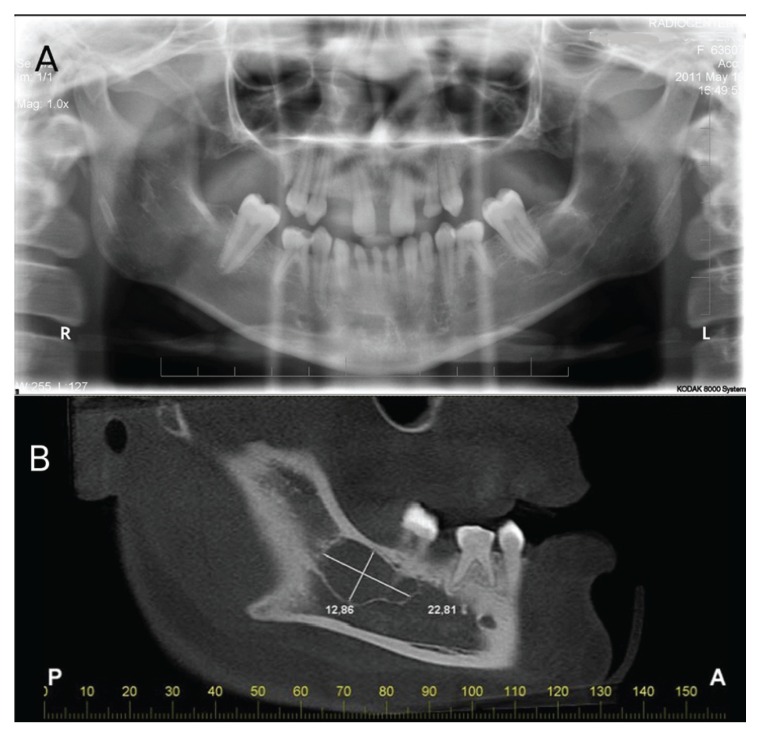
A: Panoramic radiograph of the proband showed dental agenesis, conoid teeth, taurodontism and a radiolucid image of the periapical region of the first inferior molar on the left side. B: Cone beam tomography evidence detailing the radiolucid image of the periapical region of the first inferior molar on the left side, compatible with an idiopathic cavity (simple bone cyst).
